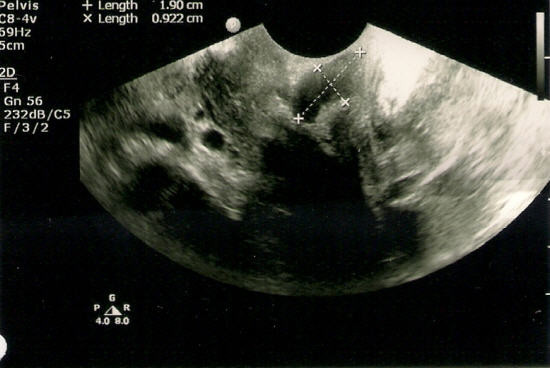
Pelvic ultrasound at the 17^th ^week of pregnancy. There was 1.9 × 0.9 cm oval hypoechoic bilobed area overlaying introitus.
